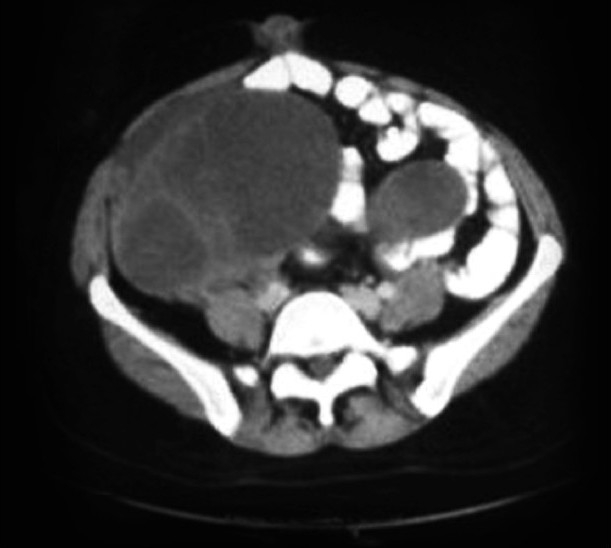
CT scan of the abdomen showing adnexal mass and skin metastasis
